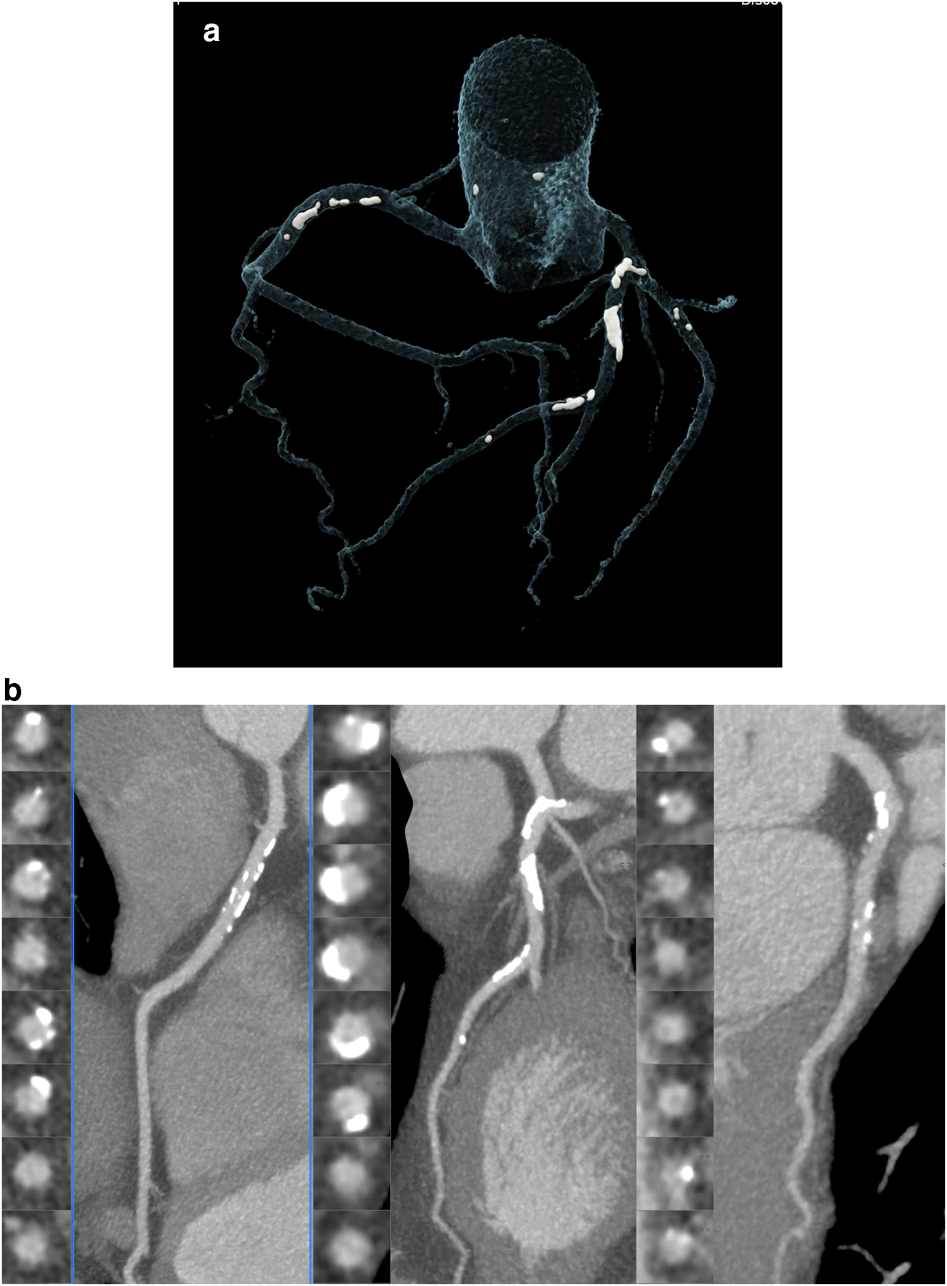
A 57-year-old-male with 1 risk factor (arterial hypertension) and ESLD due to alcohol-related liver disease. CTA was performed 2 years after quitting alcohol abuse prior to liver transplantation. MELD score was 12, and Child-Pugh Stadium B and several episodes of liver decompensations were recorded. Cardiac Calcium Score was high with 527 AU. CTA showed multiple calcified plaques in the RCA; LAD and CX with less than 50% stenosis (CAD-RADS 2). VRT (left) and cMRP (right) of RCA; LAD and CX with multiple calcified but no high-risk plaque
